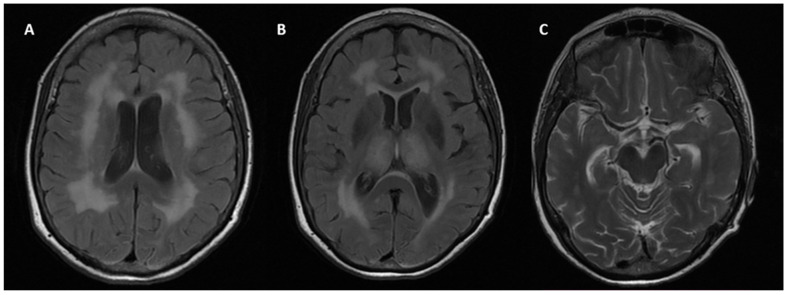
Structural MRI sequences of the patient with APP mutation presenting with Alzheimer’s disease. (A,B) Axial Flair with severe leukoencephalopathy and symmetrically increased signal intensity in the thalamus (C) Axial T2-weighted MRI image showing hippocampal atrophy.
